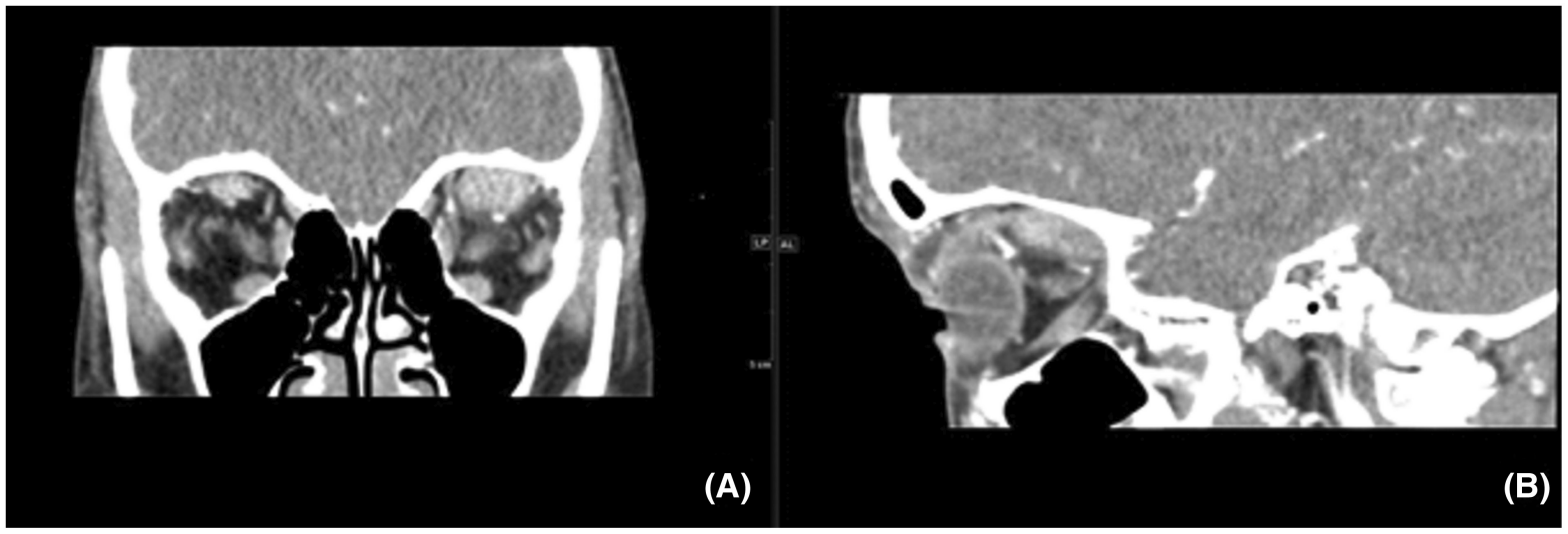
Contrast‐enhanced CT scan of the orbit reformatted images on coronal (A) and sagittal (B) images show diffusely enlarged left superior rectus muscle with intense homogenous enhancement with enhancement of its tendinous insertion.
